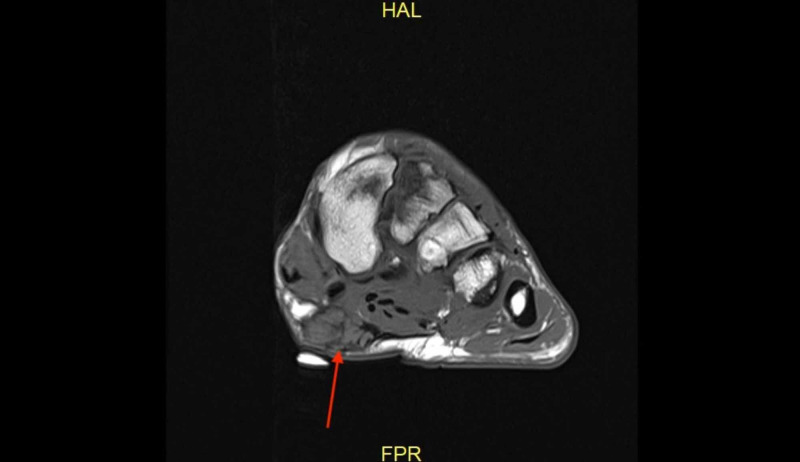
Case 2 MRI showing the axial T1 weighted image of the foot demonstrating a plantar fibroma of the midfoot, demarcated with a skin marker on the plantar aspect of the foot.
